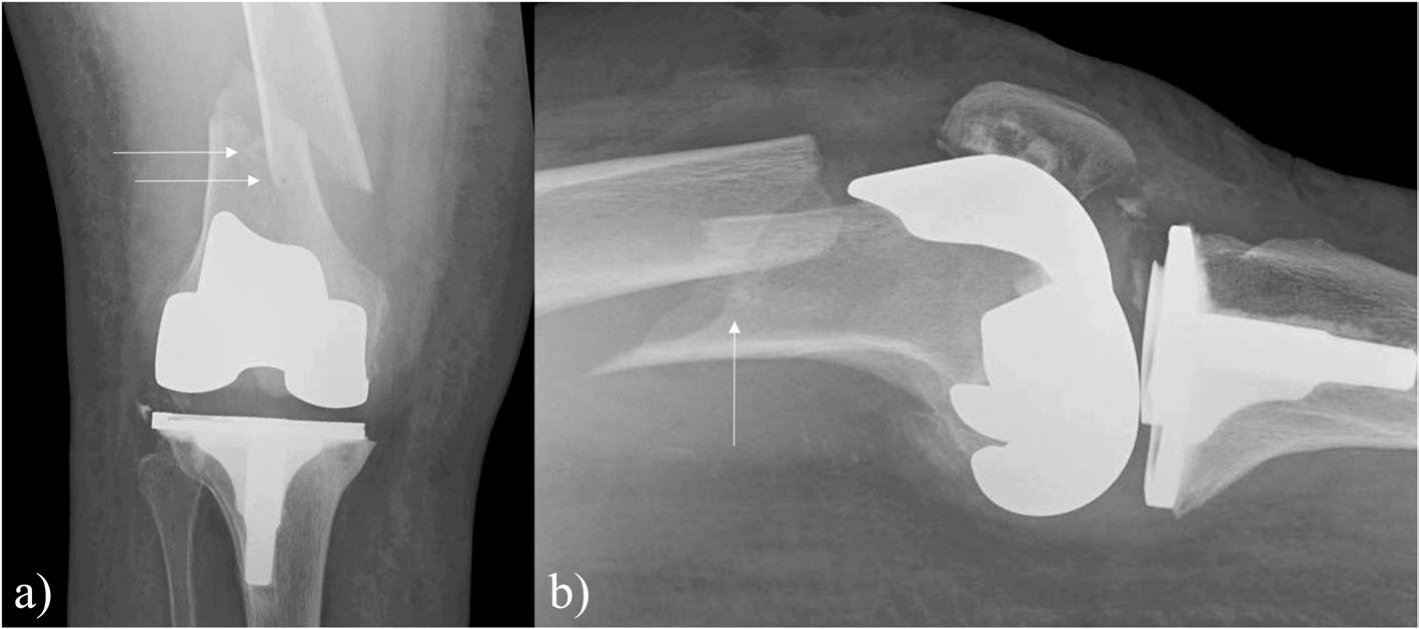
a AP and b lateral radiographs of a right distal femur postoperative pin-site periprosthetic fracture in a 75-year-old female following robotic-assisted total knee arthroplasty with the large pin diameter system. Arrows demarcate pin tracts, with the fracture line seen passing through the proximal pin tract
